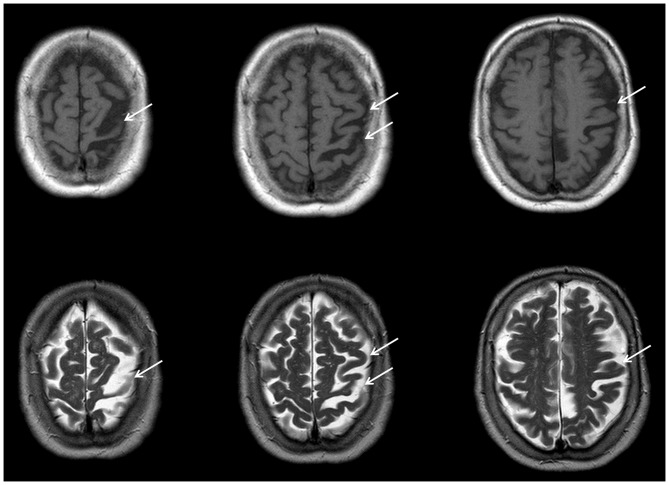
Transaxial MR imgages of the patient. T1-weighted (first row) and T2-weighted (second row), showing distinct atrophy of the left superior frontal and parietal cortices (arrows) involving predominantly the primary somatosensory (Brodmann areas 1, 2, 3) and primary motor cortex (Brodmann area 4). MR data were acquired with a 3 T Magnetom Trio Siemens scanner (3D-SPGR, TR = 24 ms, TE = 8 ms).
